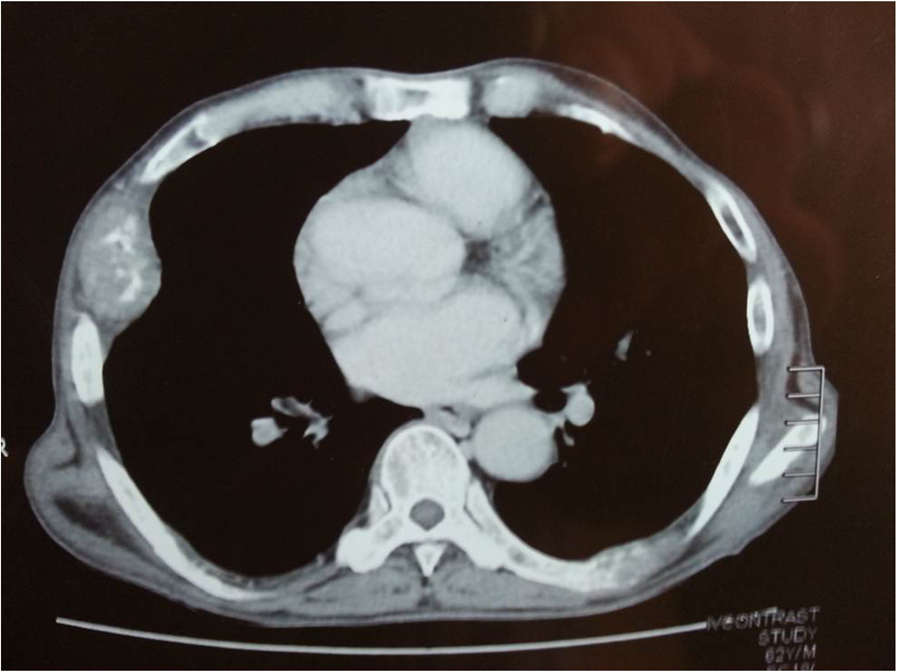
Computed tomography of thorax showed erosion of left 7th & 8th rib and soft tissue mass involving right side of chest wall
